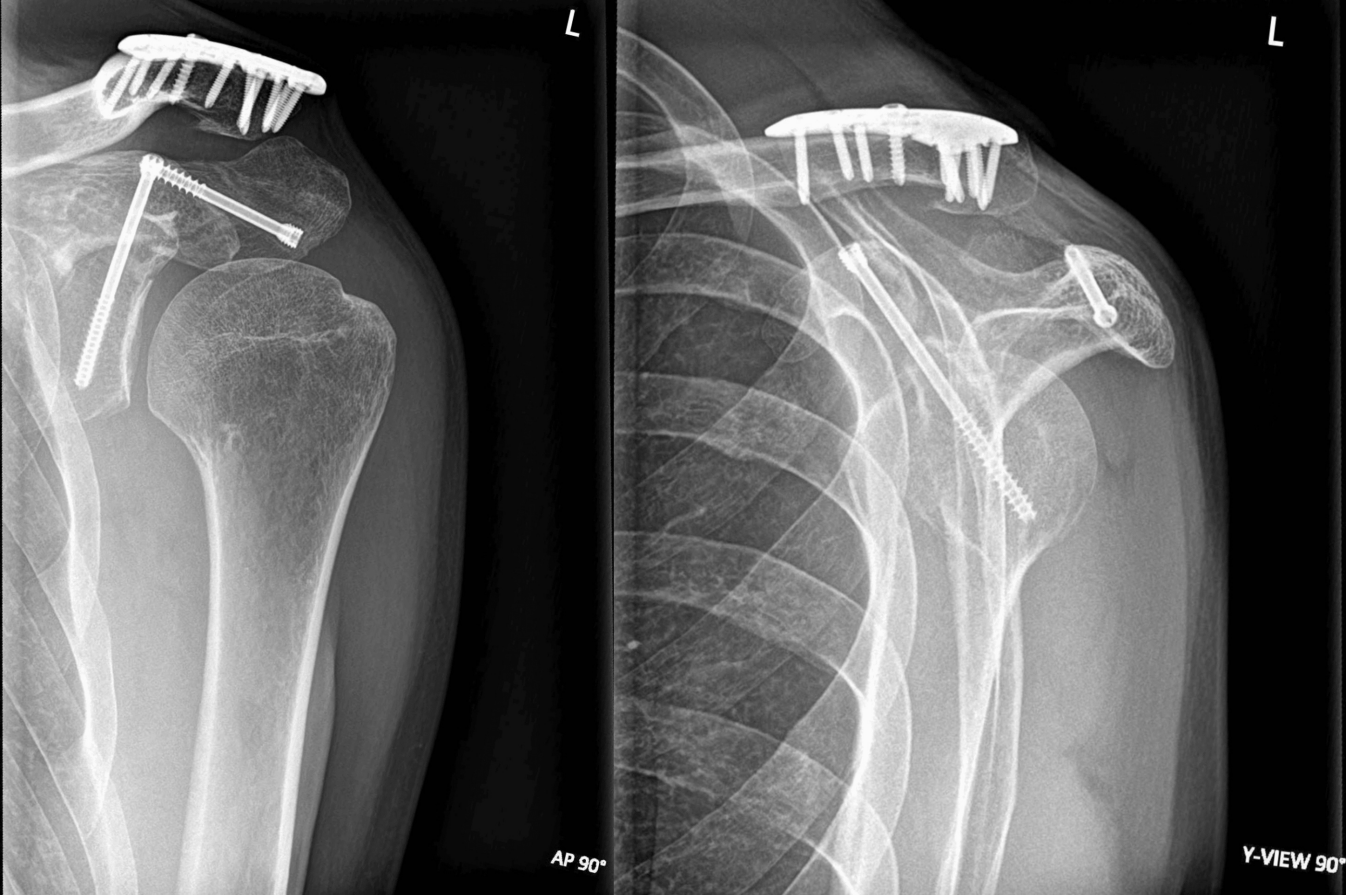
Six-week postoperative radiograph of the shoulder girdle A six-week postoperative radiograph in the coronal view demonstrating progressive bone healing in the shoulder.
